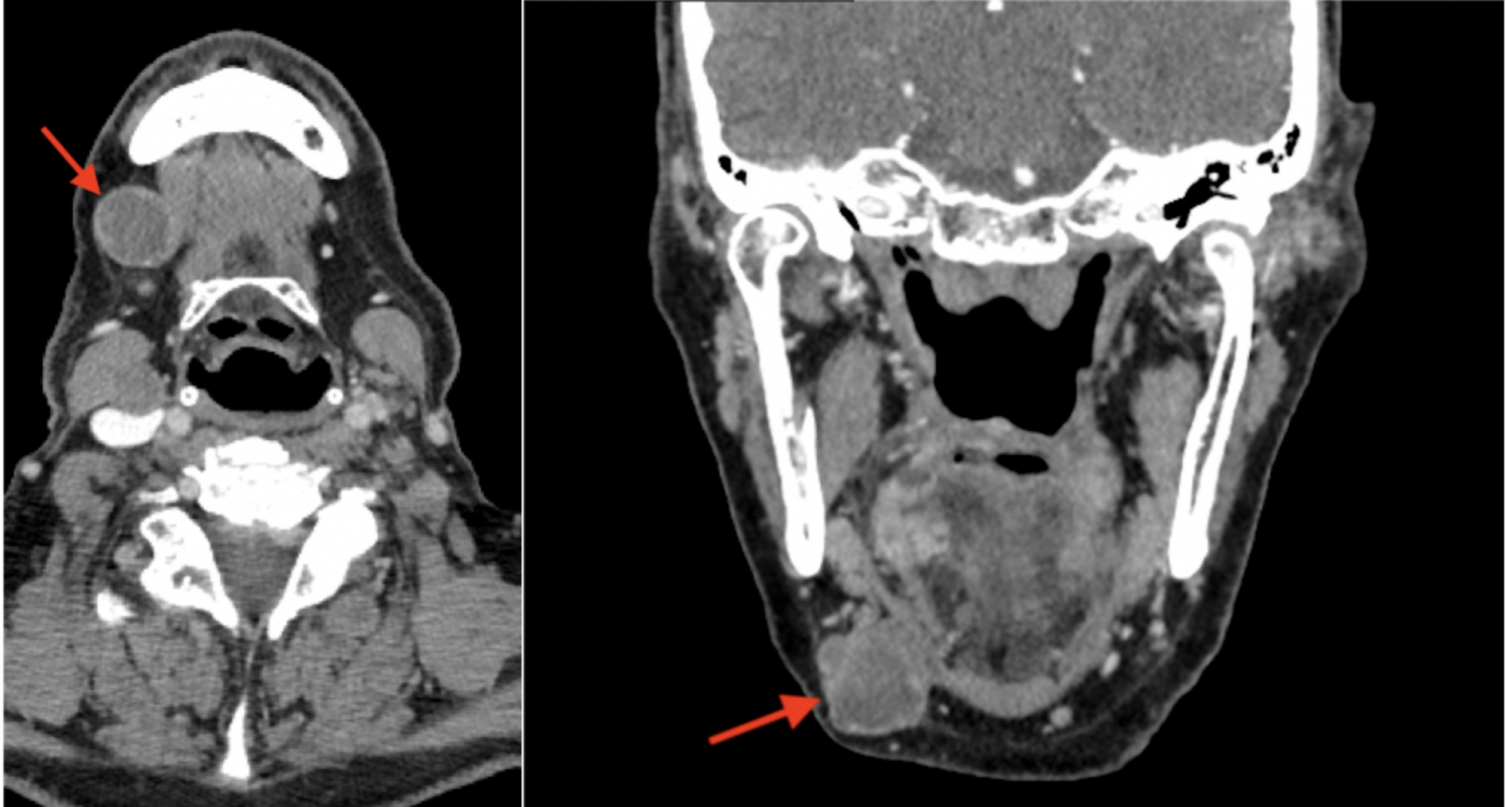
Head and neck CT showing hypodense rim enhancing soft tissue lesion in the right submandibular space (red arrows).
